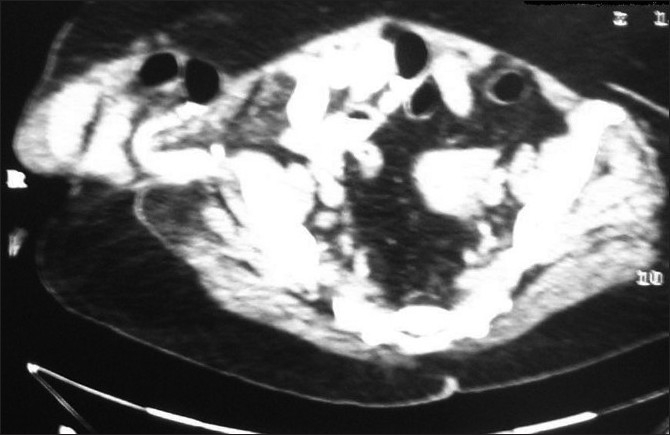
Contrast enhanced computed tomography of the abdomen showed herniation of the bowel loops through the abdominal wall defect into the subcutaneous tissues
